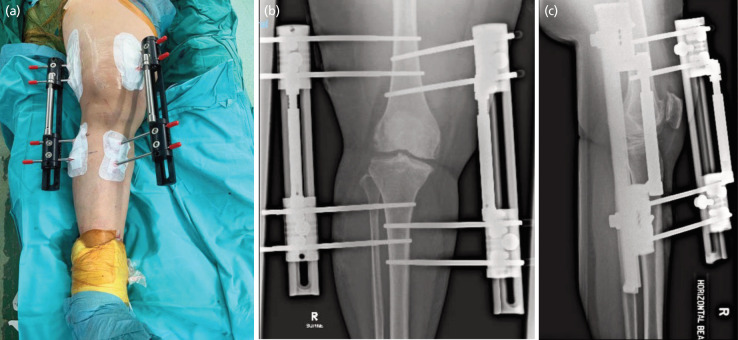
Clinical photographs and post-operative radiographs of knee distraction. (a) Post-application of KJD device. (b and c) KJD with external fixators.
